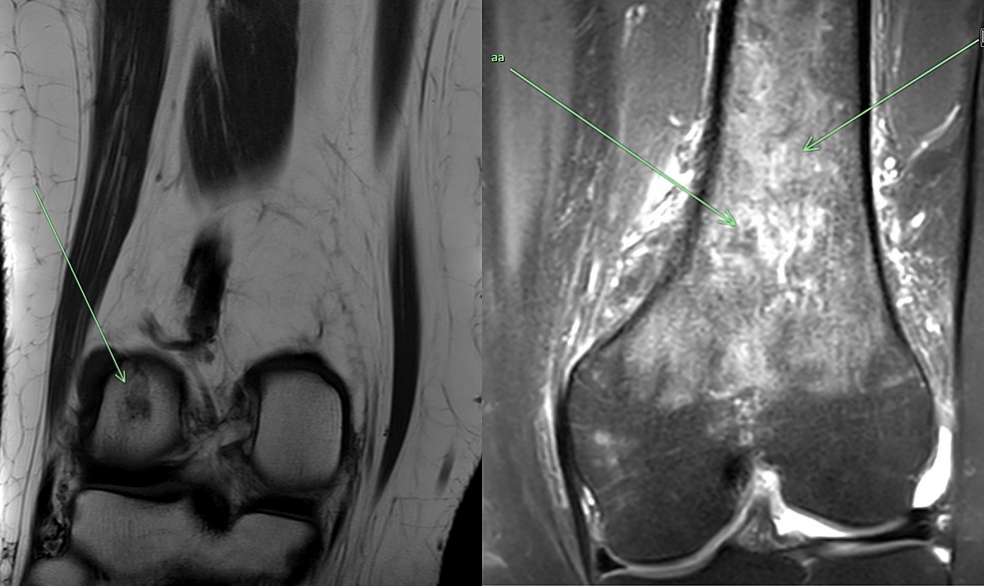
Image on the right with green arrows: MRI of the knee joint showing circumferential periosteal edema involving bilateral distal femoral meta diaphysis, which is suggestive of medullary infarcts. Image on the left with a green arrow: MRI of the right knee showing an area of bone marrow edema-like signal that represents avascular necrosis at the lateral femoral condyle.
